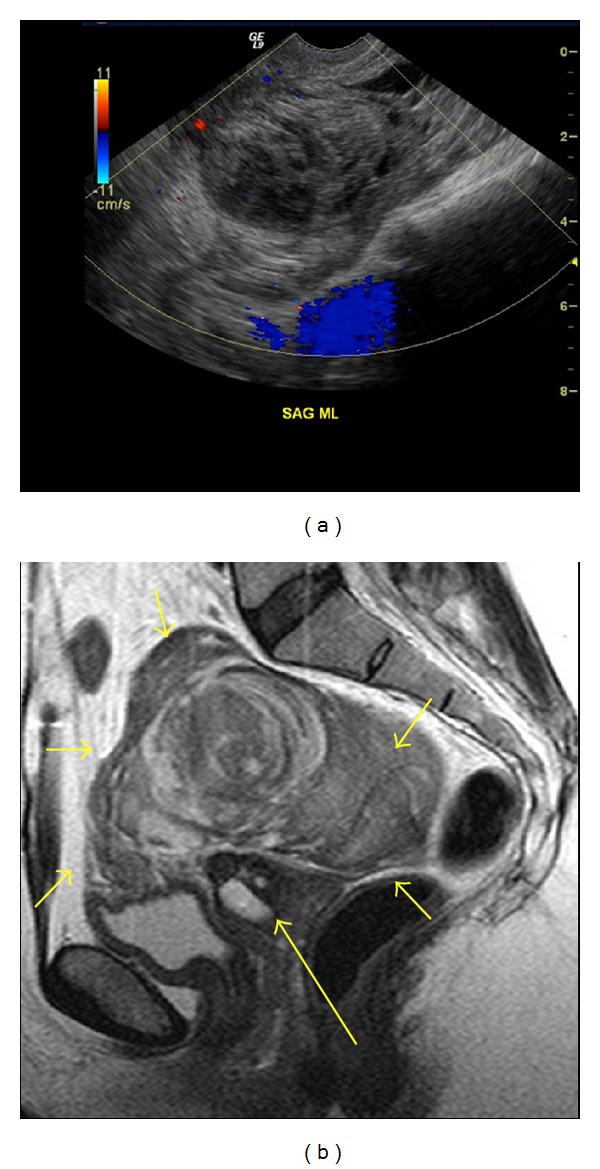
Image (a) is a transvaginal sagittal image with Doppler through the midline pelvis demonstrating a large central heterogeneous mass in the pelvis which does not show internal flow likely representing an organizing hematoma. Image (b) is a T2 weighted sagittal MR image through pelvis showing a large central pelvic hematoma (small arrows). A prominent cervical stump containing a nabothian cyst (large arrow) is noted posterior to the bladder.
